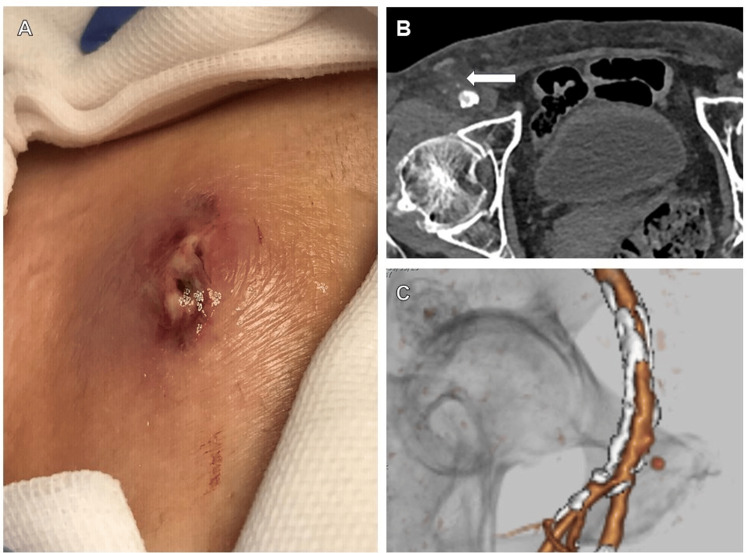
Photographs and CT images of the puncture site in case 2. A: Photograph of the puncture site in the right femoral artery. Redness, swelling, and pus are present. B: Contrast-enhanced CT image of the puncture site. A soft tissue shadow with mild contrast enhancement can be seen around the puncture site. C: Contrast-enhanced 3D CT image. Although it was small, with a diameter of 3 mm, a pseudoaneurysm is shown in front of the puncture site. CT, computed tomography
